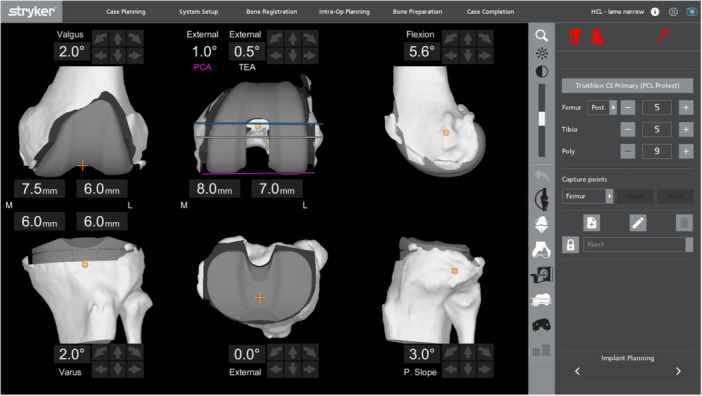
Bone pillar. Preoperative CT‐based planning of the reported case, showing implant positioning and planned bone resections.
